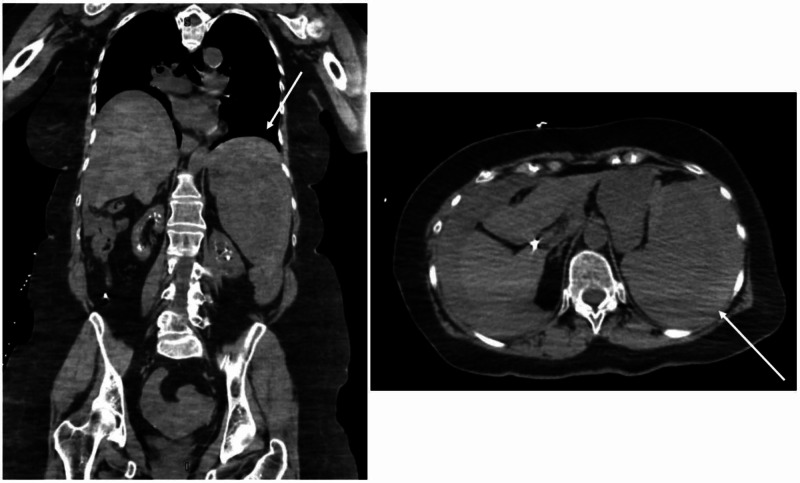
Initial CT chest, abdomen, and pelvis w/o IV contrast Significant for dense, abnormal fluid within the abdomen and pelvis Right) Coronal view; Left) Axial view IV: intravenous
